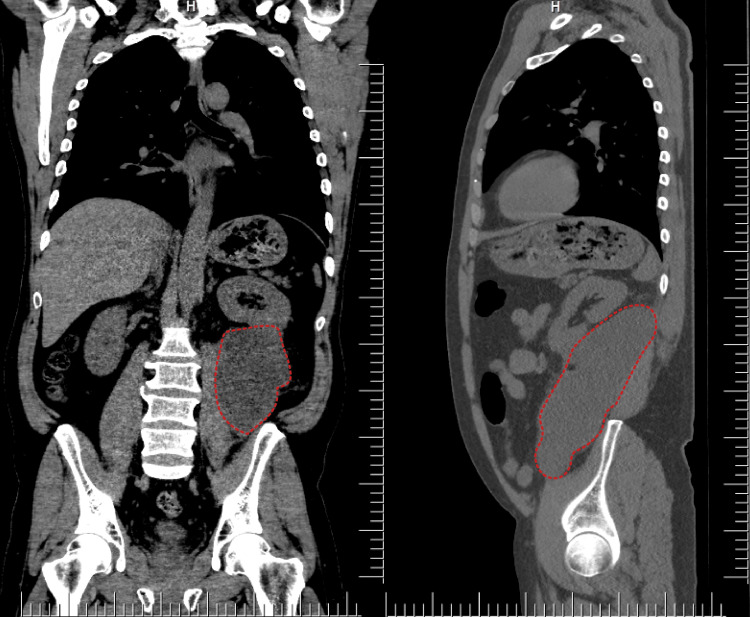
Abdominal CT image with contrast. Left image: sagittal view; right image: coronal view. Red dotted lines represent the retroperitoneal hydatid cyst.
